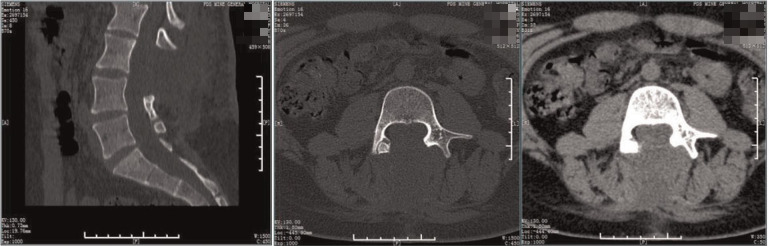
Postoperative CT. The spur was excised and the laminae of the L3-5 segments were not fused.
